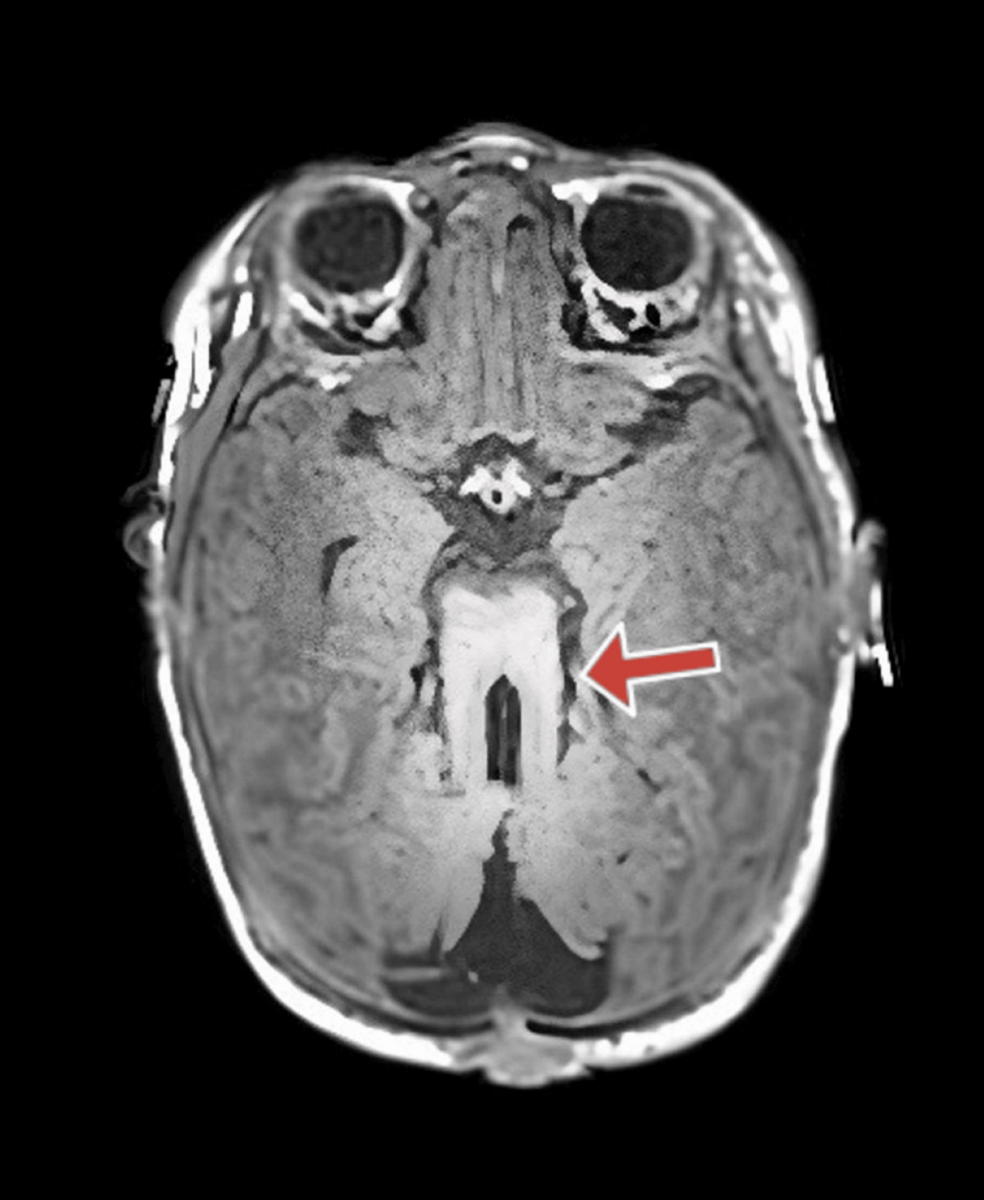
Axial MRI image showing the molar tooth sign characteristic of Joubert syndrome
